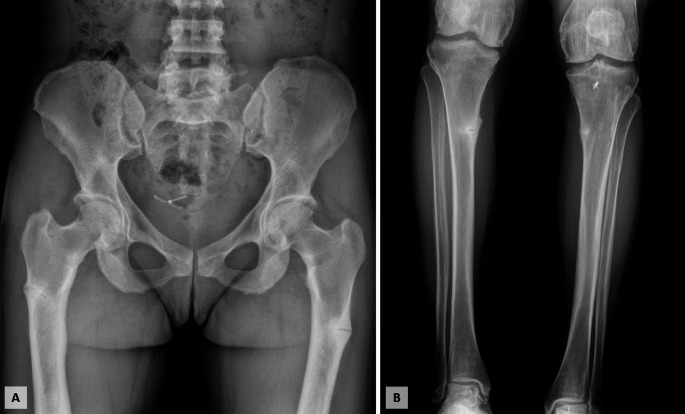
X-rays of the lower limbs showing multiple pseudofractures involving the femurs (A) and tibias (B)
